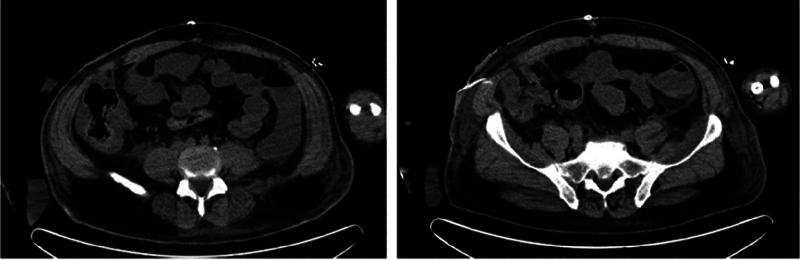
Nonenhanced abdominal computed tomography scan showing right colitis.
